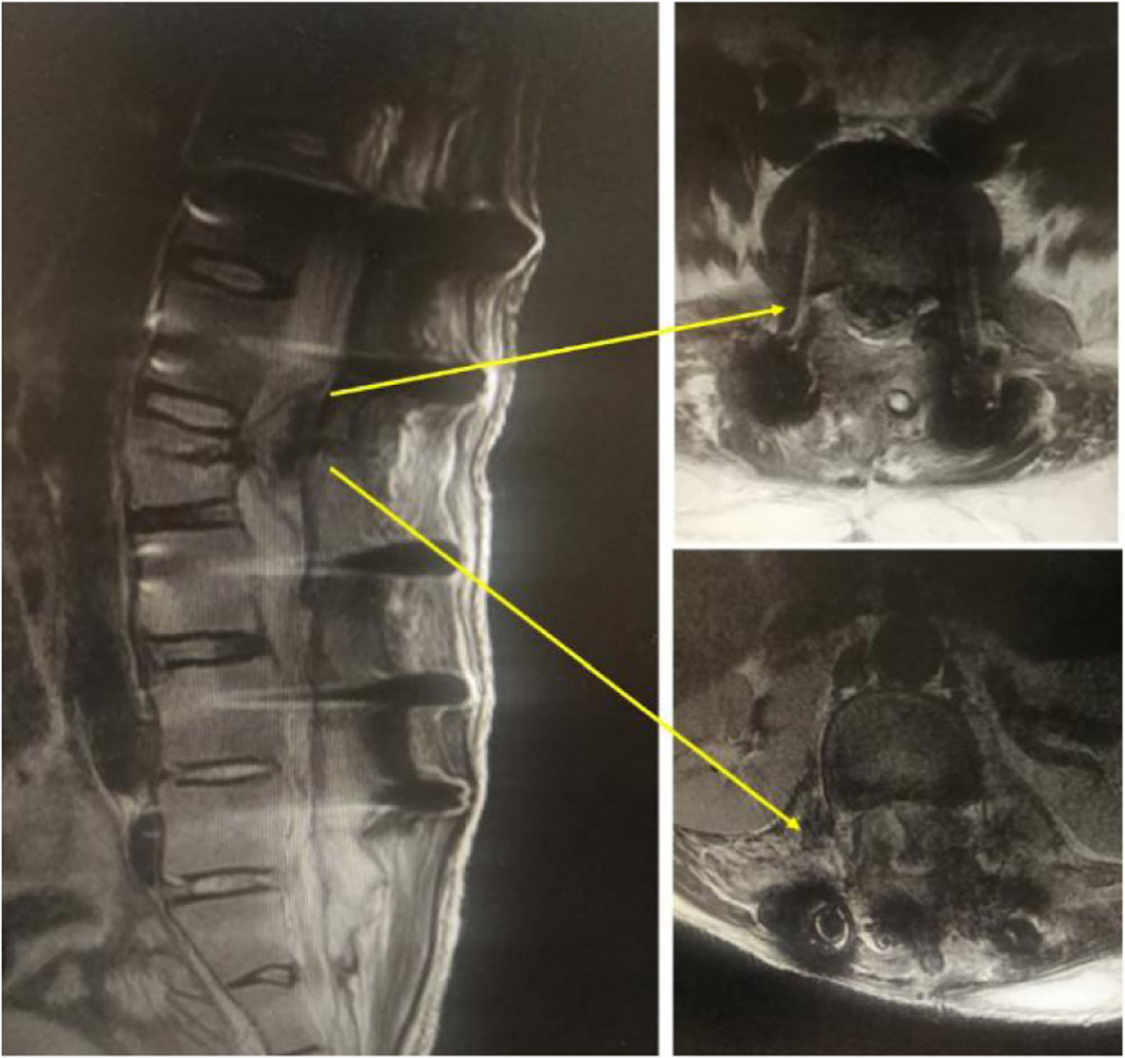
A 26-year-old male experienced numbness in his right lower extremity and had grade 1 hip flexion muscle strength a day after a PLF and an L2 pedicle subtraction osteotomy due to ankylosing spondylitis. The MRI showed an epidural hematoma behind the L2 vertebral body. The patient’s symptoms were relieved, and his muscle strength recovered after the hematoma was removed
